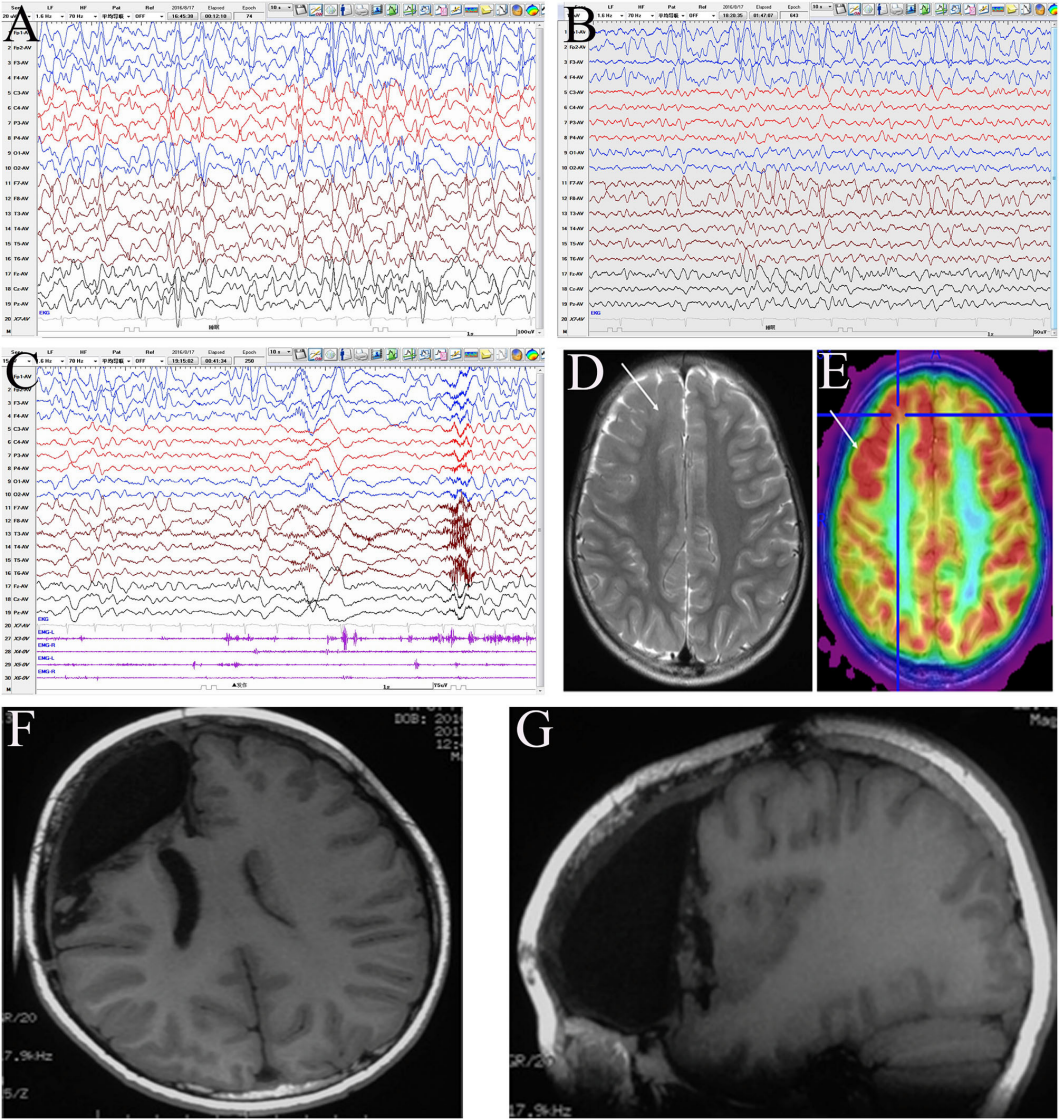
Evaluation of a male patient, 5 years old with onset age at 1 year and 6 months and clustered asymmetric epileptic spasm, which was obvious in the left upper limb. The patient had been prescribed several anti-seizure medicines, including adrenocorticotropic hormone, valproic acid, topiramate, and levetiracetam, with no success. The patient had mental and motor developmental delay and no obvious hemiplegia. Interictal electroencephalogram (EEG): hypsarrhythmia (A), with intermittent right frontal regional continuous discharge (FP2, F4, and F8) (B); ictal EEG: fast rhythm dominated on the right hemisphere (C). MRI: focal cortical dysplasia (FCD) in the right frontal lobe (D), with boundary-clear focal hypermetabolism on positron emission tomography (PET) (E). According to the presurgery evaluation and intraoperative electrocorticography, the resection scope included the right prefrontal lobe, anterior cingulate cortex, frontal central operculum, and anterior insula (F,G). Pathology: a few dysmorphic neurons (FCD IIA) in the ACC and malformation of cortical development in other areas. The patient has been seizure-free for 3 years and shows mild mental and motor progression.
